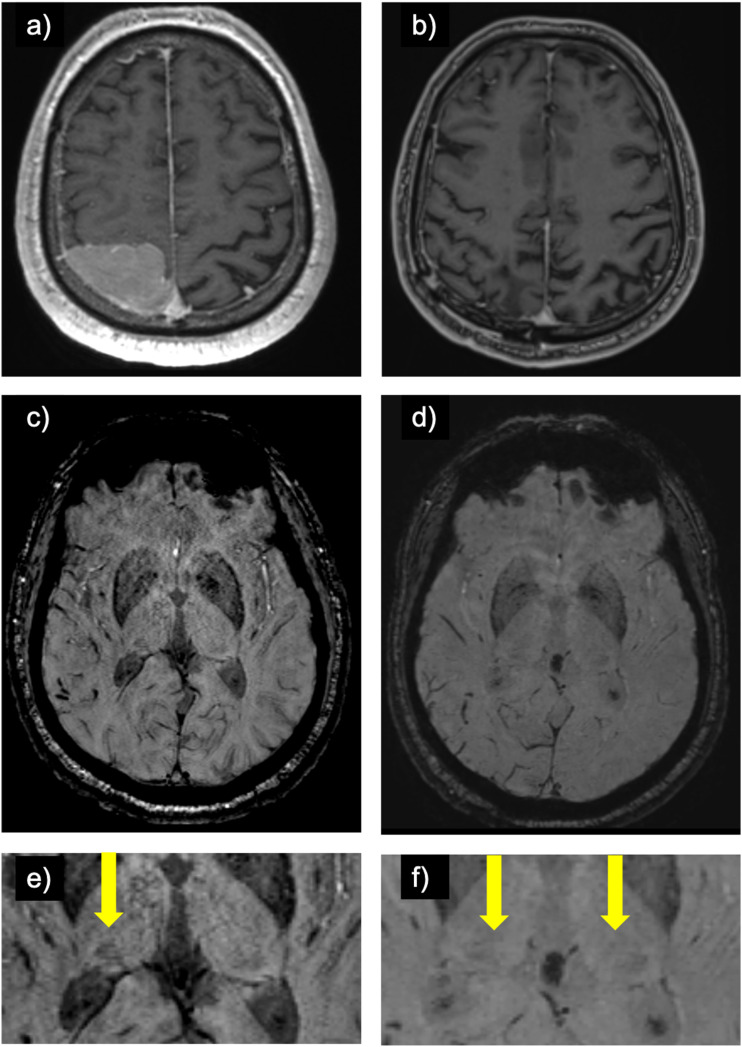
Post glioblastoma resection development of the hypointense pulvinar sign: (a) contrast-enhanced T1-weighted image of a patient with a left temporal glioblastoma. (b) symmetrical hypointensity of the pulvinar on susceptibility weighted imaging on early post-resection MRI (“equal”; arrows on zoomed-in image c)) which evolved to a left-sided hypointense pulvinar sign upon further follow-up (d; arrows on zoomed-in image e)).
